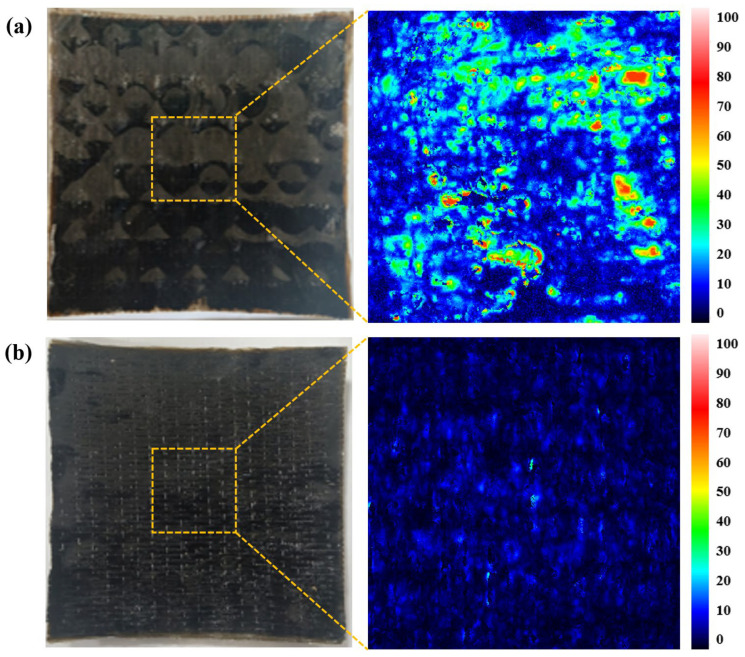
Ultrasonic C-scan results for (a) components with significant dimples and (b) dimple-free components.
